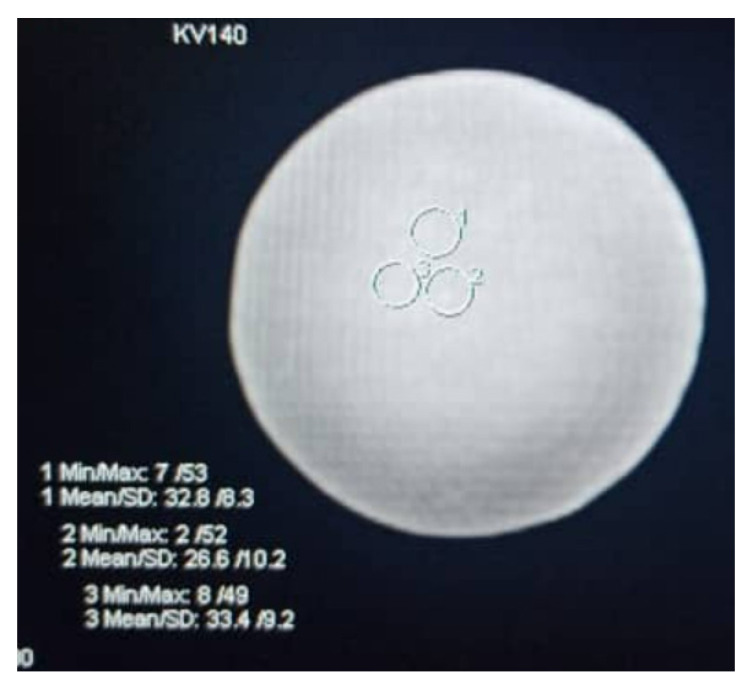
Example of objective image quality analysis to assess the CT number (mean) and image noise (SD) by putting the circular region of interest (ROI) in the middle of the sample.
